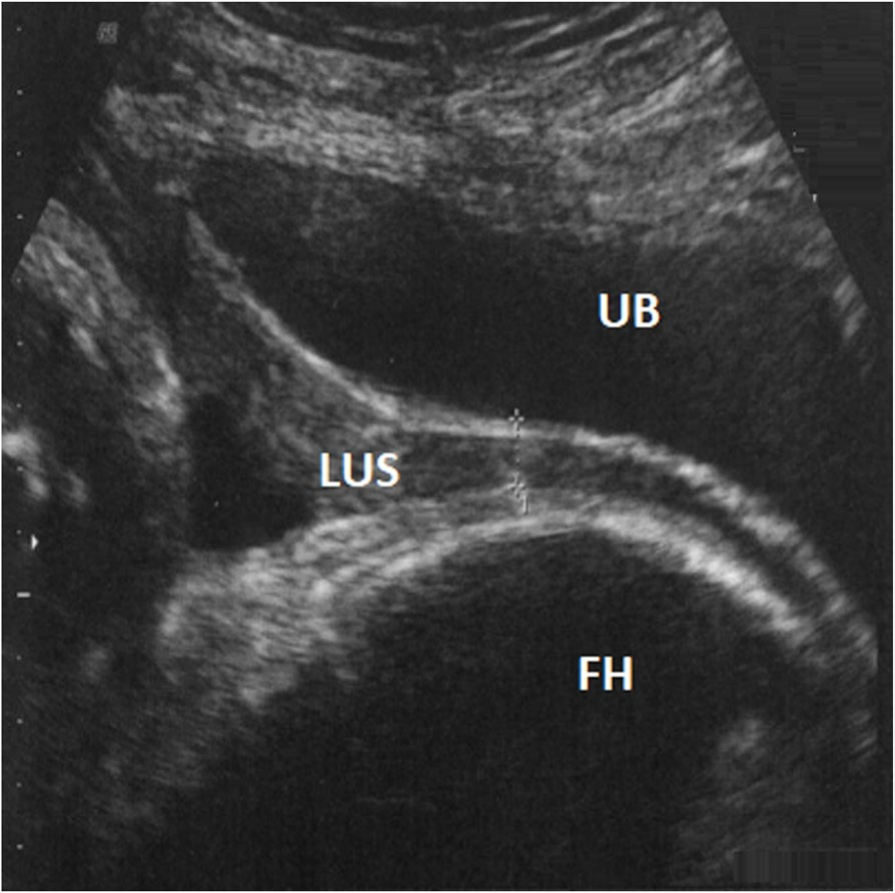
Trans vaginal ultrasound during first stage of labor with empty bladder, measuring the Lower Uterine Segment (LUS) and the LUS with posterior UB wall, which shows a normal LUS thickness (2.5 mm). M: myometrium, F: fetus head
